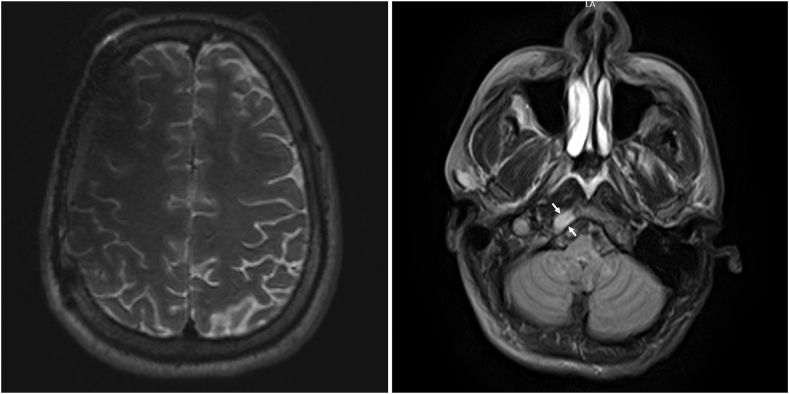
Magnetic resonance imaging findings 3 months after the resection of the right frontal lesion. (Left) The right frontal lesion was no longer seen. (Right) The right occipital bone lesion persisted but remained unchanged in size (thin arrows).
